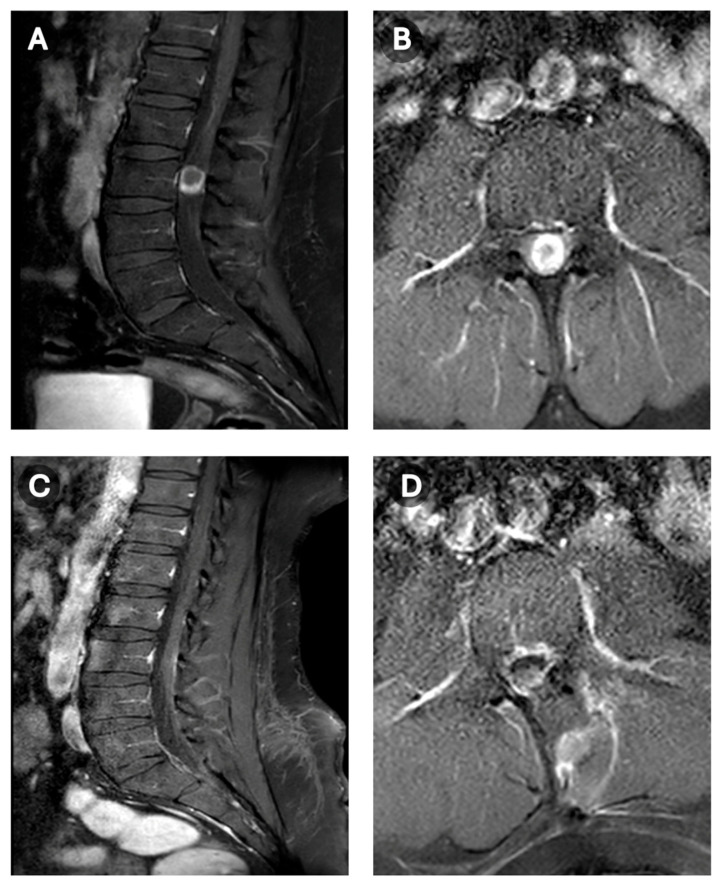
Preoperative and postoperative MRI findings of Case 9. Sagittal and axial preoperative contrast-enhanced T1-weighted images (A,B) demonstrate an intradural extramedullary lesion at the L3 level with heterogeneous enhancement. Postoperative images (C,D) confirm gross total resection.
